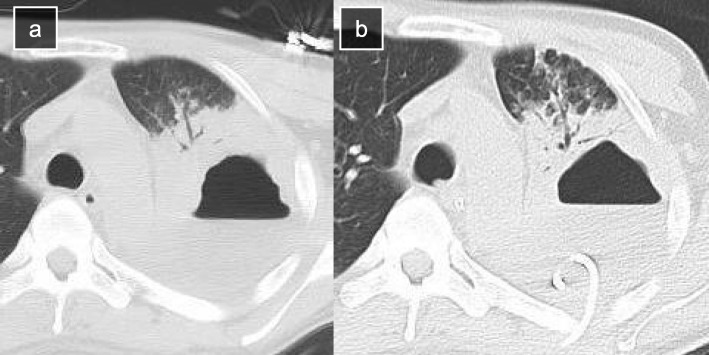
Computed tomography findings before and after initial percutaneous drainage. a A large abscess with an air-fluid level in the left upper lobe. b Residual abscess content due to incomplete initial drainage
